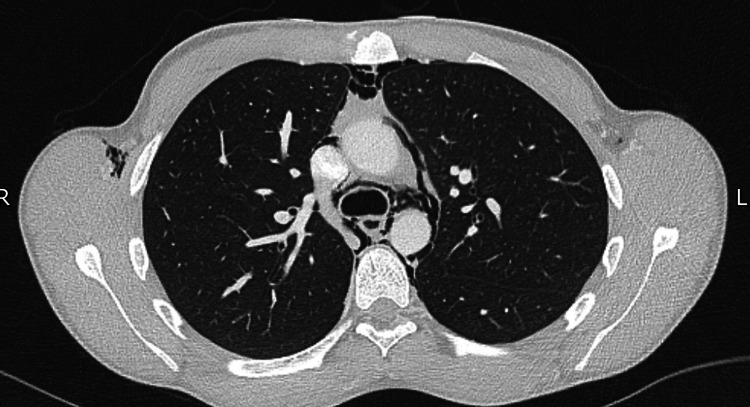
CT of the thorax image on day 1 showing pneumomediastinum.
